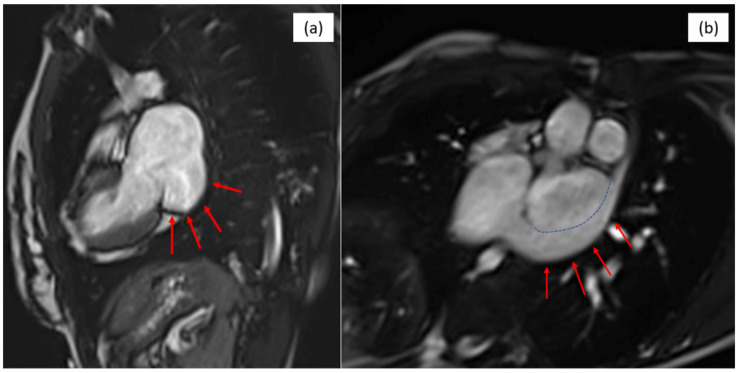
MRI images of (a) the unroofed coronary sinus (red arrows) and (b) left superior vena cava (red arrows) and demarcation between the coronary sinus and the left superior vena cava (blue dashed line).
